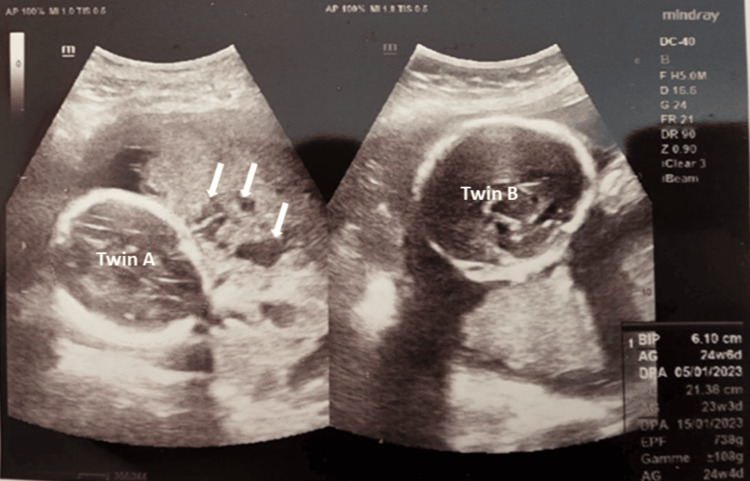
Preoperative Obstetric Ultrasound Findings in Twin Pregnancy With Suspected Placenta Accreta Spectrum Preoperative obstetric ultrasound at 24 weeks + 6 days. Left panel (Twin A): anterior placenta previa with irregular intraplacental lacunae (arrows) suggestive of placenta accreta spectrum (PAS). Reliable assessment of invasion depth was limited by the emergency setting and complexity of the twin gestation. Right panel (Twin B): normal fetal cranial biometry. BPD: biparietal diameter; GA: gestational age; EFW: estimated fetal weight; PAS: placenta accreta spectrum.
